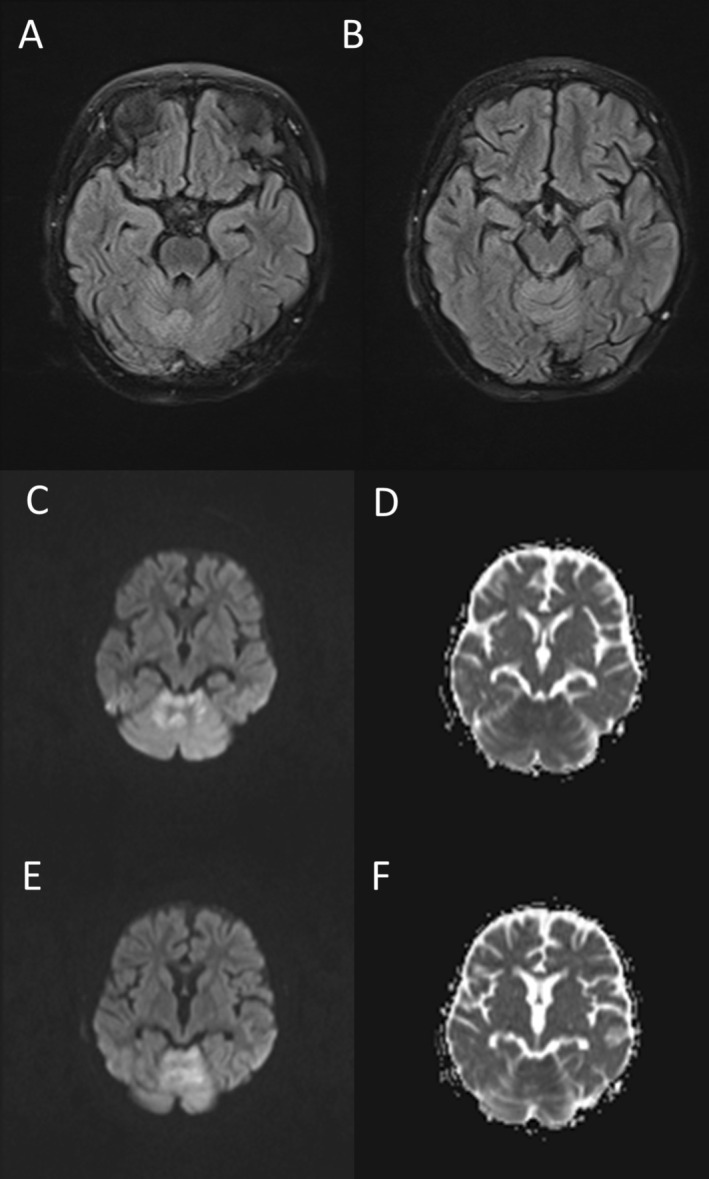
First MRI: Fluid attenuated inversion recovery (FLAIR). MRI showing hyperintensities in cerebellum (A, B). Restricted diffusion in cerebellum (C–F).
